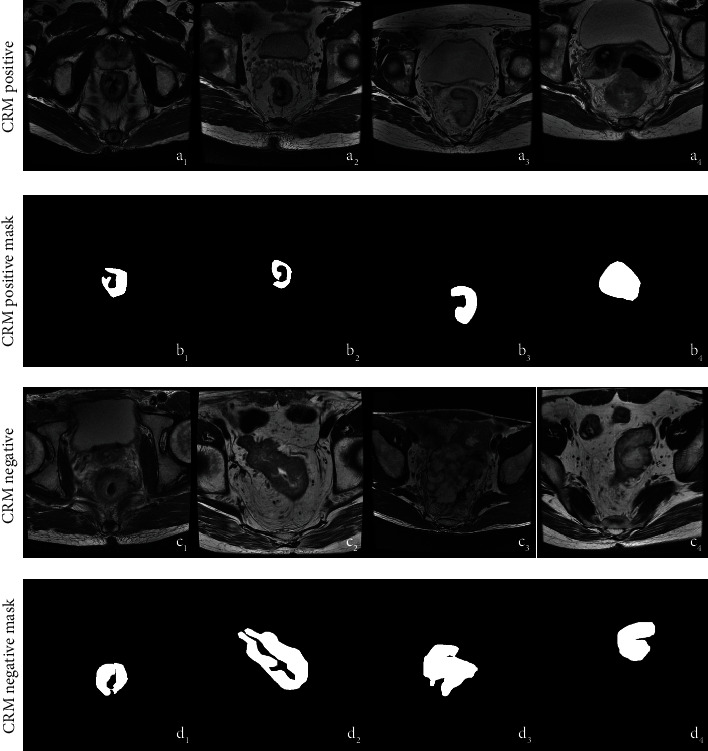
Examples of colorectal cancer in MRI images. (a1–a4, c1–c4) Are the original image slices from the MRI DICOM series; (b1–b4, d1–d4) are the tumour region mask labelled by doctors.
